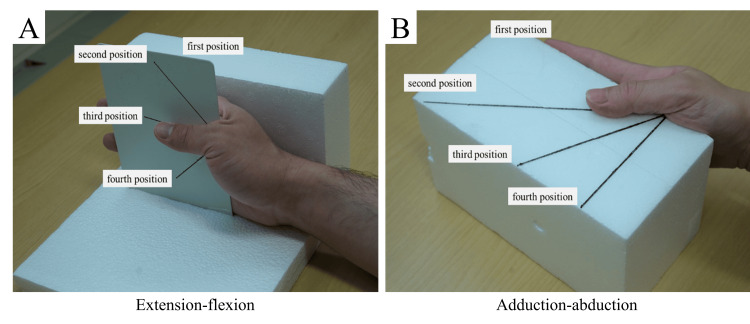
Photograph of the self-made assistive device used for CT imaging of thumb flexion and abduction. This device enables vertical and horizontal movement with respect to the palm. A shows the device for extension-flexion, and B shows the device for adduction-abduction. This figure was created by the authors.
